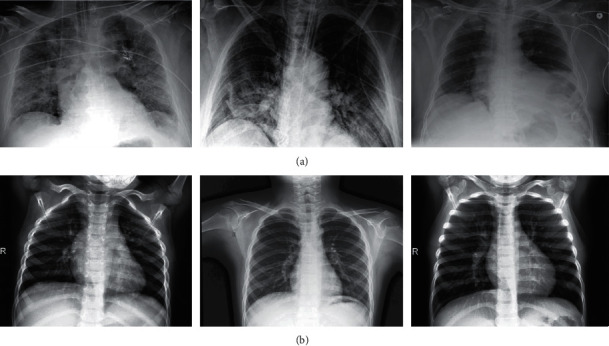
Partial sample of the COVID-19 dataset. (a) A chest X-ray of COVID-19. (b) A chest X-ray of healthy people.
